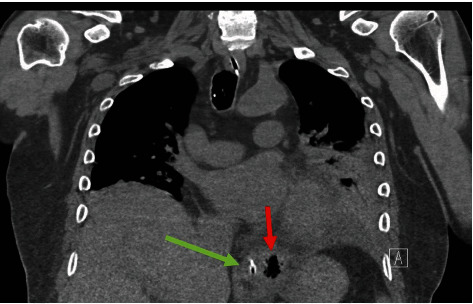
Noncontrasted coronal CT images showing a pocket of air in the mediastinum left lateral to the esophagus (red arrow) consistent with esophageal perforation. Green arrow identifies an NG tube within the esophageal lumen.
